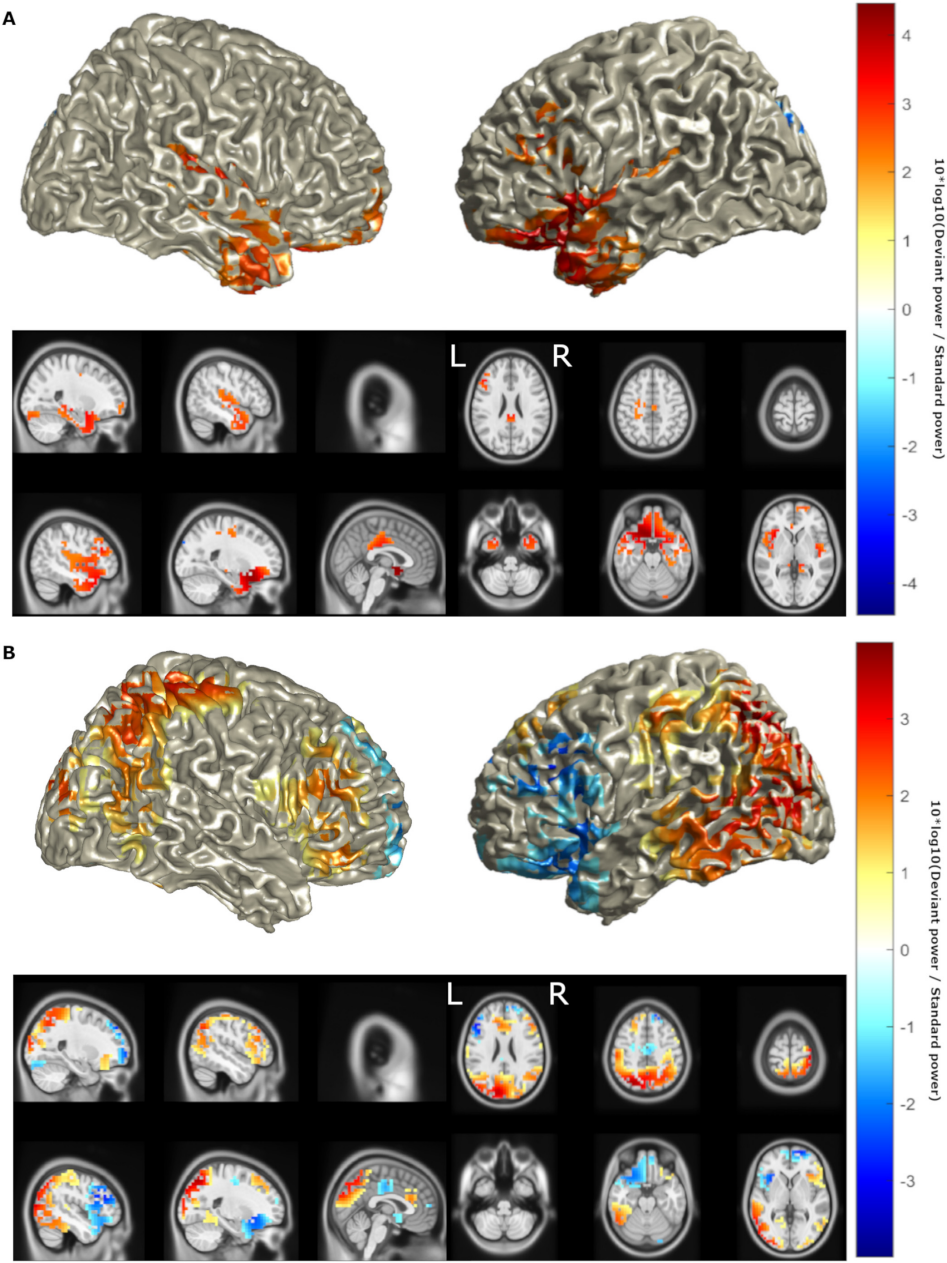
ELORETA identified a pattern of decreased activity in the left superior temporal and inferior frontal sources, and an increase in activity in posterior areas. Location of MMN sources with (a) top 50% of power (10*log_10_(Deviant power / Standard power)) in healthy controls and (b) power differences >25% of maximum between ALS patients and healthy controls as determined by eLORETA. Red denotes increase in power, blue denotes decrease in power. Axial MRI views are from above (L-Left, R-Right).
